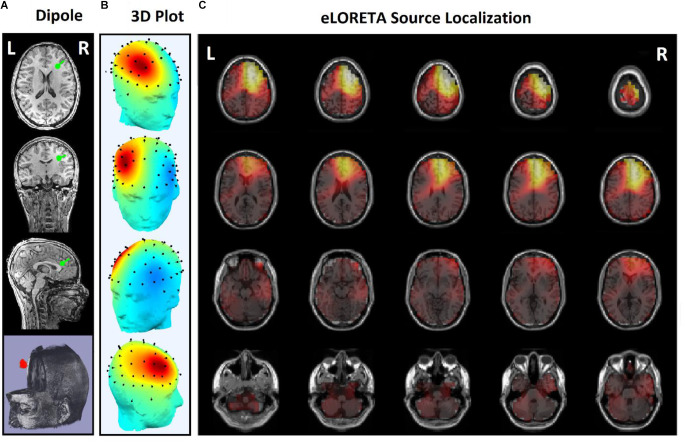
Source localization of the rTMS stimulation foci in right prefrontal cortex (R-DLPFC) for a responder subject. (A) Dipole coordinates of the identified focus in right side on a default MRI. (B) Position of the foci identified by ICA on the surface of the skull. (C) Source localization in right DLPFC by eLORETA.
